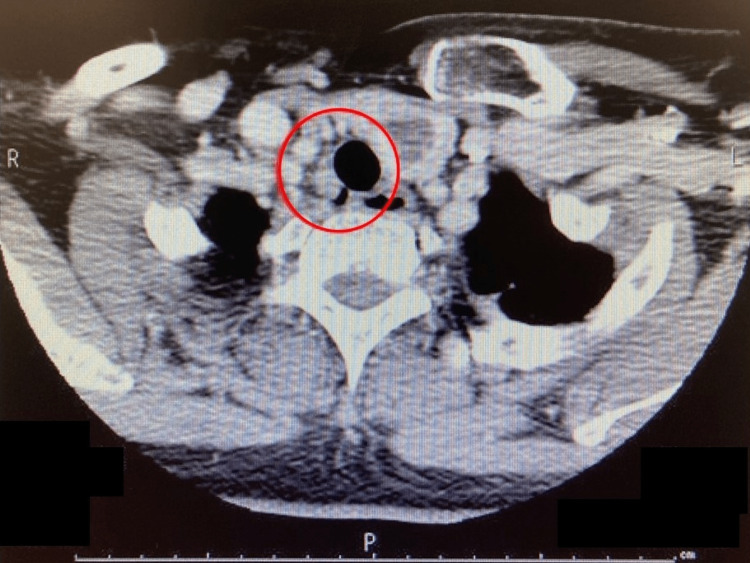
Plain chest computed tomography indicating irregularity in the right tracheoesophageal groove
